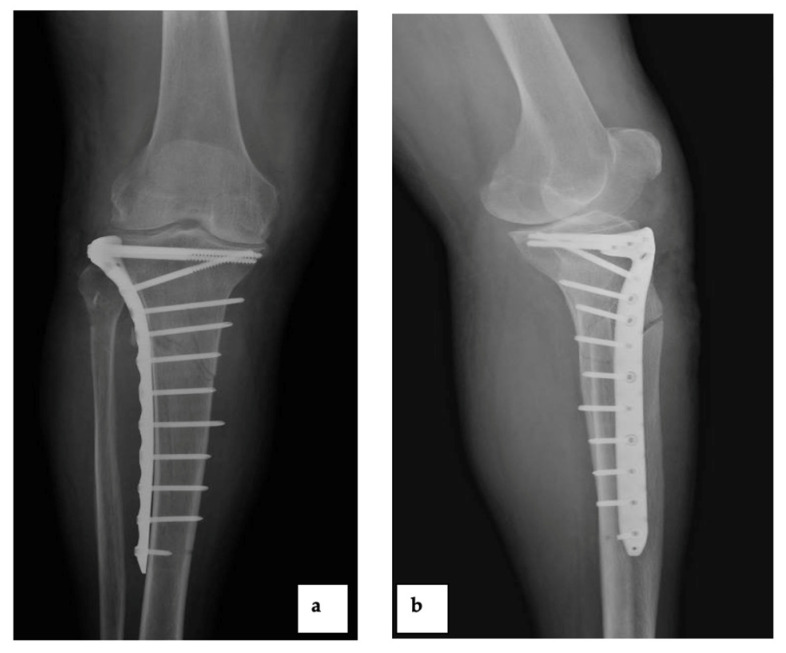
The plain radiograph showed the infected tibial plate ((a): anteroposterior view and (b): lateral view).
